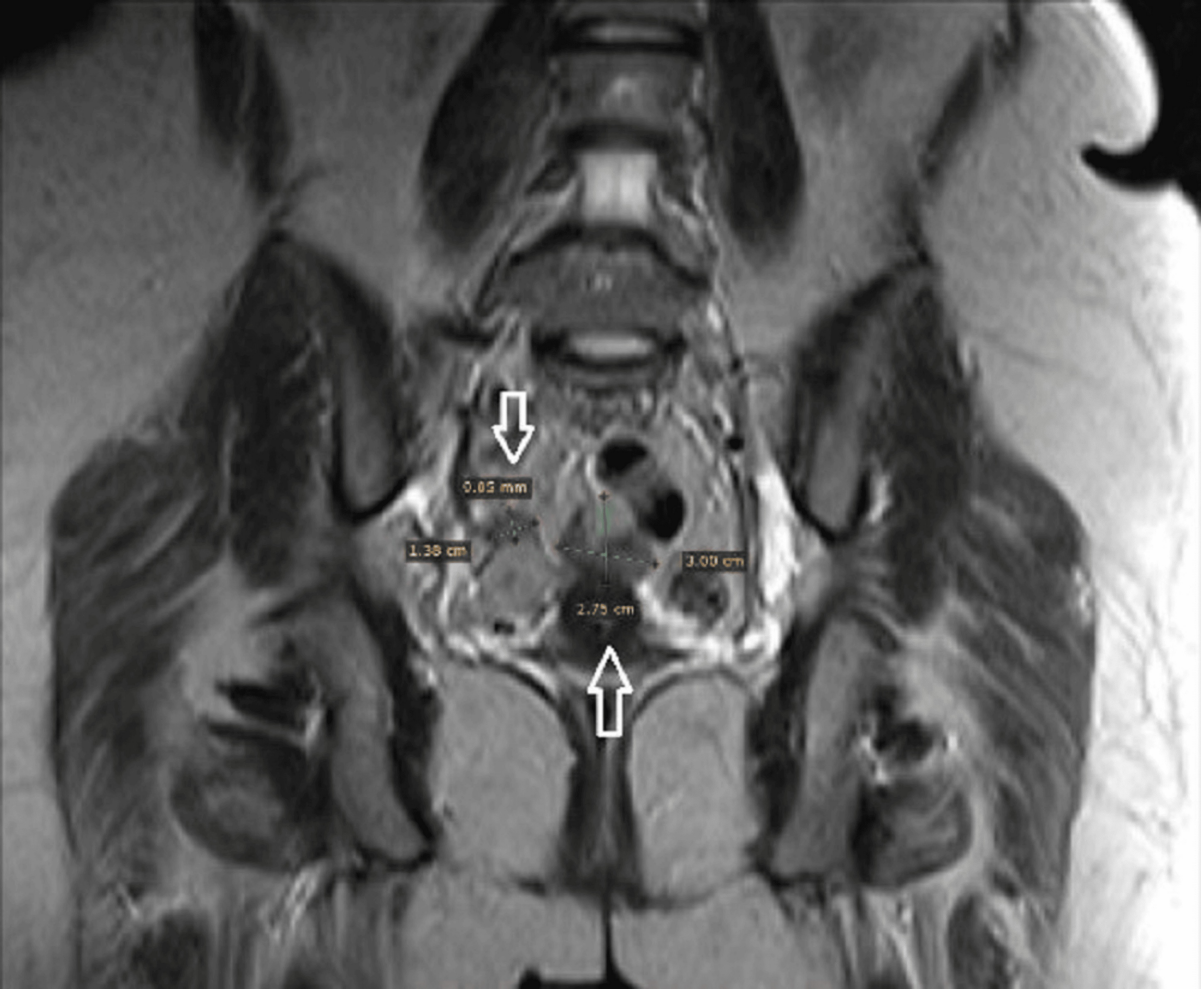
MRI showing multiple pelvic nodules (arrows)
